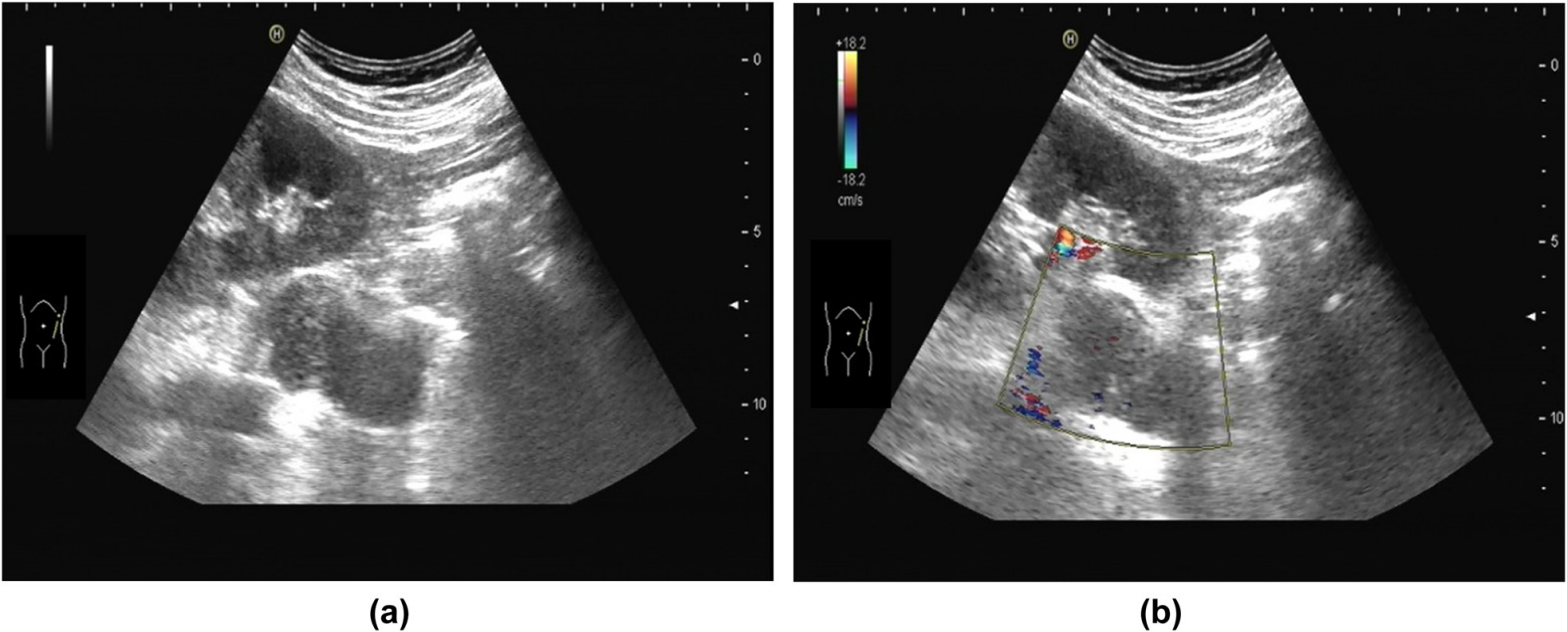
(a) Ultrasonic image: mass in the left retroperitoneum with an irregular shape and uneven internal echo. (b) Ultrasonic image: the CDFI shows no blood flow signal of the mass.
